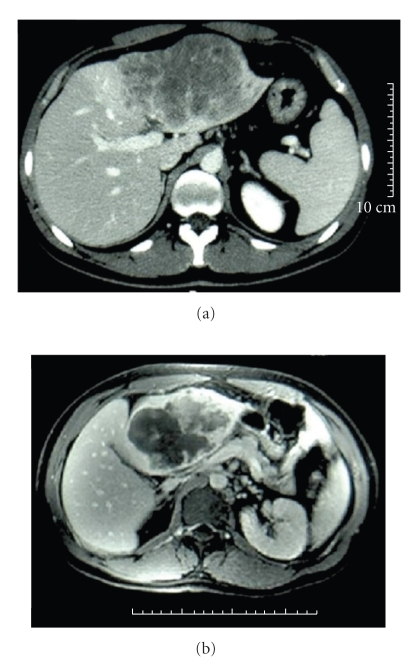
CT-scan (a) and the Magnetic Resonance Imaging (b) showed a voluminous heterogeneous tumour occupying the left liver. The injection of contrast showed a late vascularisation.
